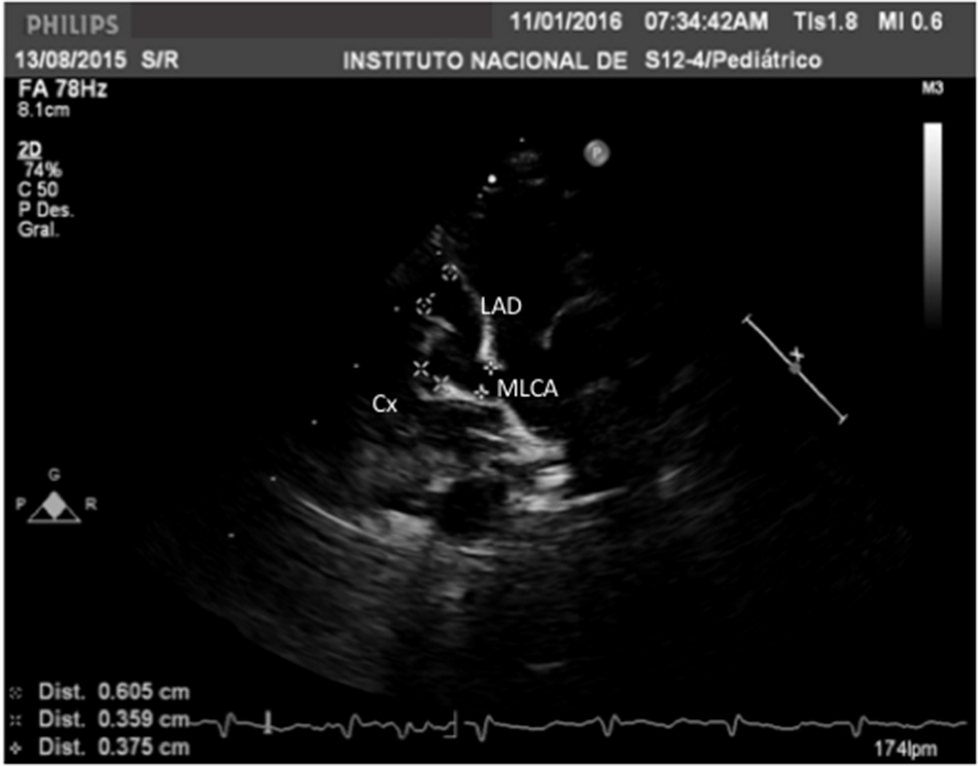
Echocardiogram in a modified long-axis parasternal view in a 2-months-old boy with Kawasaki disease diagnosed after 18 days of the onset of fever. Weight 4.7 kg, height 55 cm, BSA 0.27 m^2^. With a large coronary artery aneurysm in the main left coronary artery with z-score of +7.70; a giant coronary artery aneurysm in the left anterior descending artery with a *z*-score of +17.29 and a large aneurysm of the circumflex artery with a *z*-score of +8.99.
